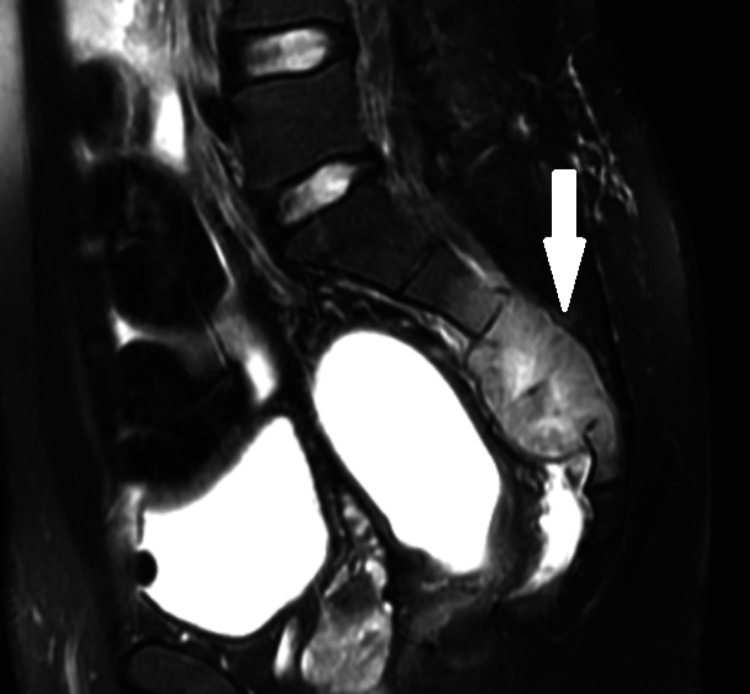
MRI of the sacrum showing S3 and S4 vertebrae with a paraspinous mass (white arrow) MRI: magnetic resonance imaging.
